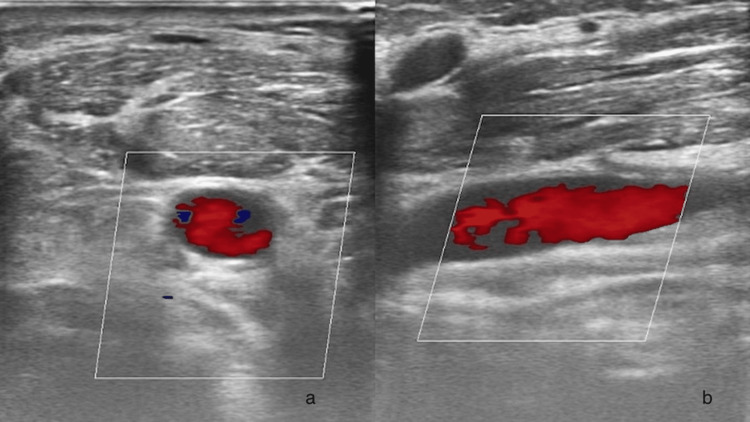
Duplex ultrasound verifying hemostasis after use of the closure device
